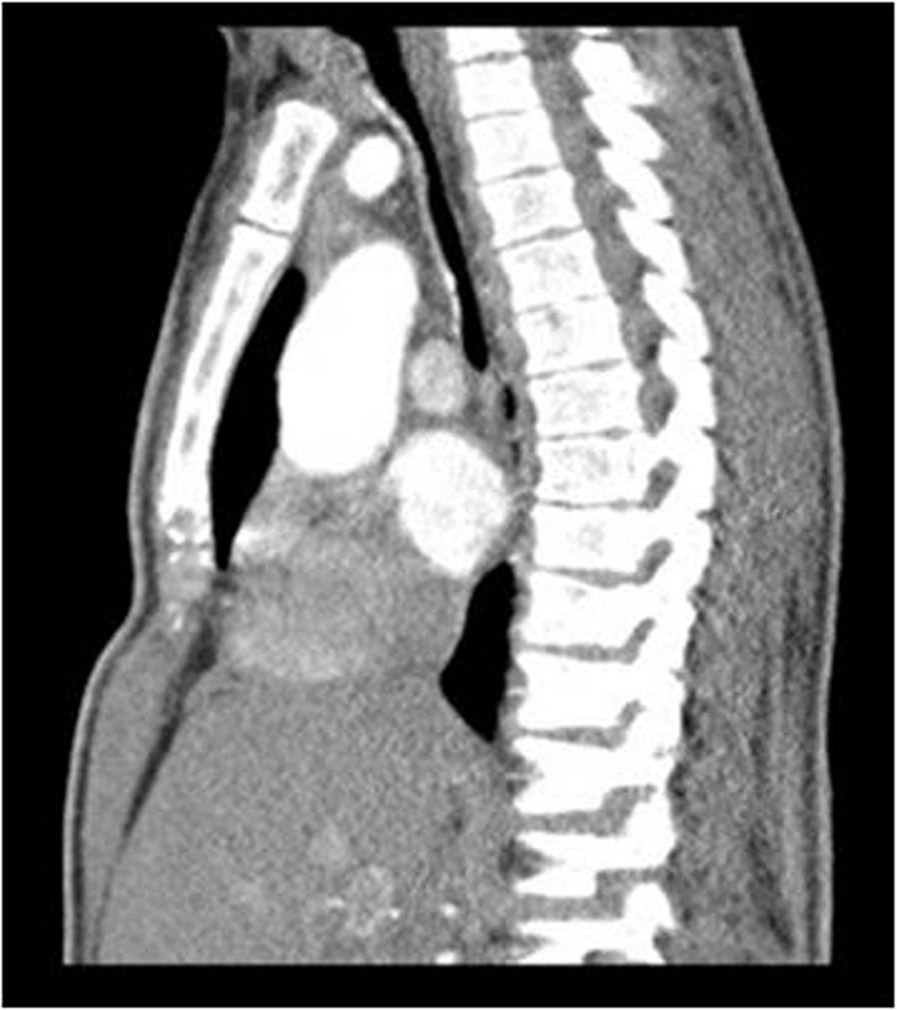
Computed tomography (CT) of the respiratory tract in MPSII patient after bifurcated-tracheobronchial stent implantation. The CT scan confirmed the correct Y-stent position and the amelioration in the dimensions of the airways at the level of the trachea and major bronchi
